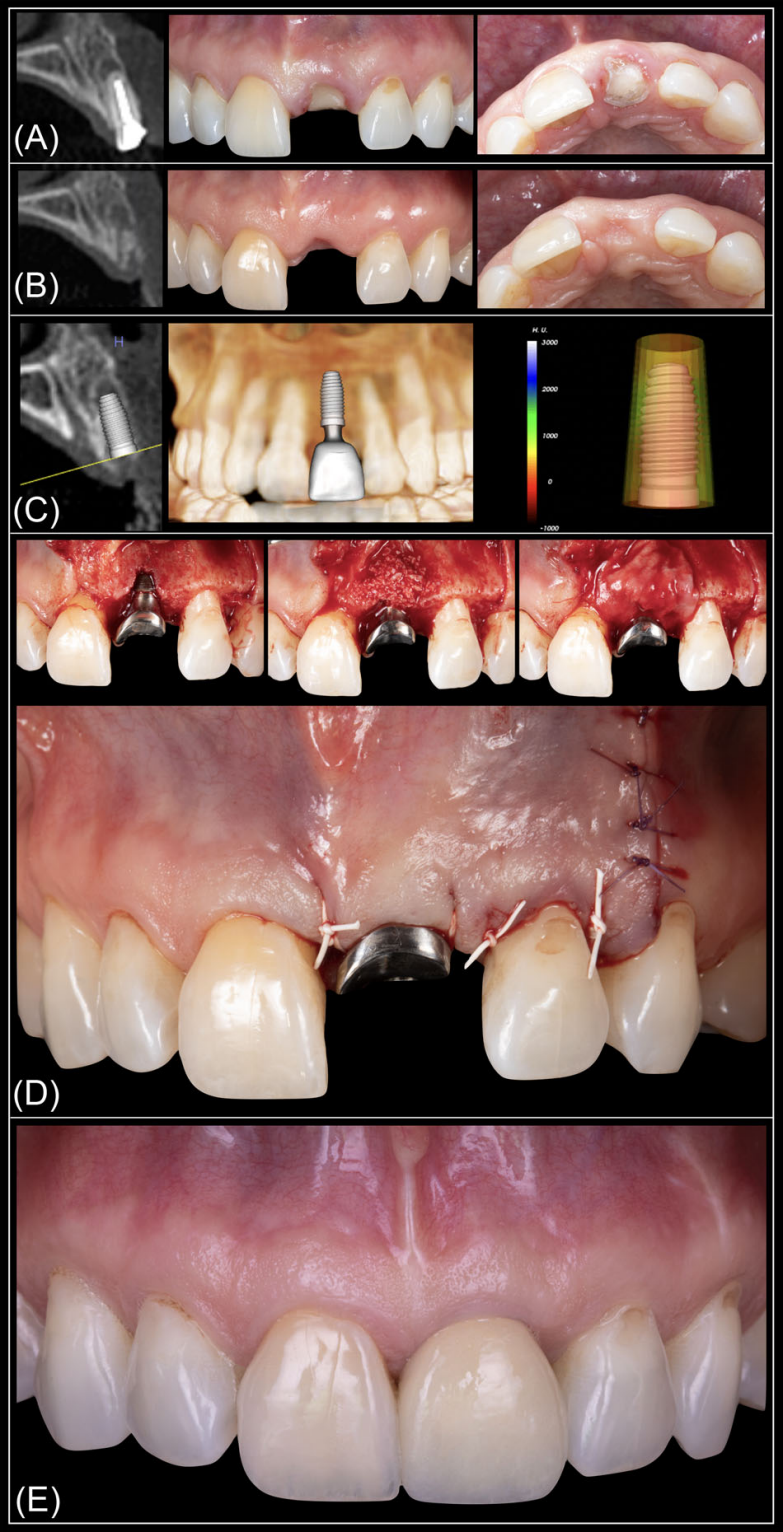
Representative case of a site presenting a thin bone phenotype. Radiographic and clinical aspect at baseline (A) and after ≈ 3 months of healing (B). Additional bone augmentation was deemed necessary upon virtual implant placement (C) and confirmed at the time of implant placement (D). Frontal intraoral view after delivery of the final restoration (E). Case restored by Dr. Christopher Barwacz, Department of Family Dentistry, University of Iowa College of Dentistry
